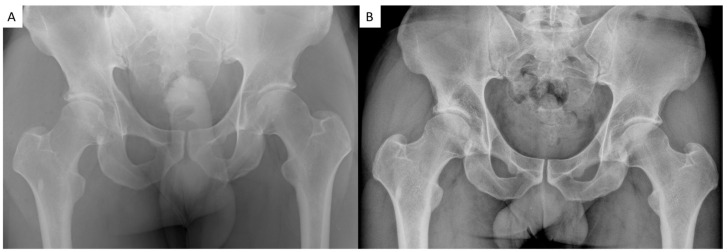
(A): immediate postoperative radiographic control; (B): 5-year radiographic follow-up showing no bone substitute migration, intra-articular extravasation, and/or local complications.
